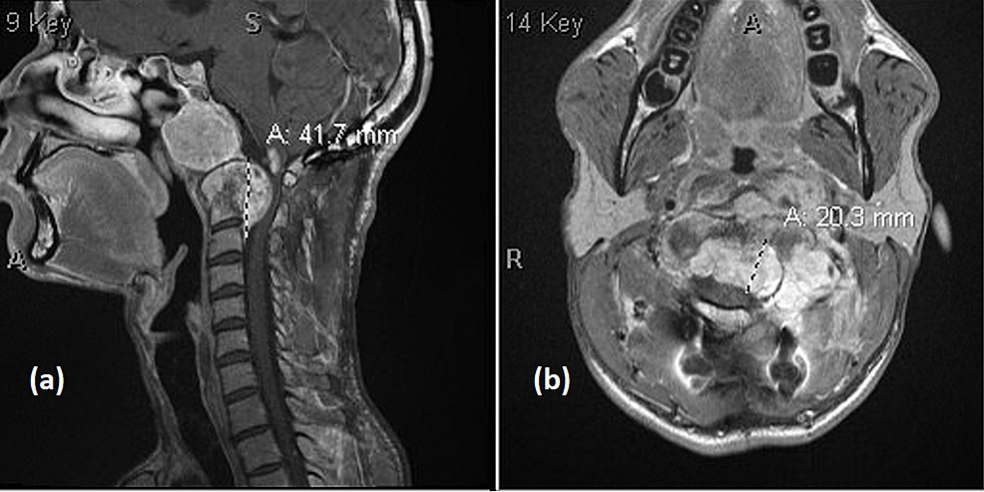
MRI spine of (a) large clival and (b) skull base chordoma. The image is showing large clival and skull base chordoma involving C1 and C2 with intraspinal epidural extension down to C3 and involvement of the left greater than right C1-C2 neural foramina. The mass effect on the cervicomedullary junction is greater in the left ventral cord than in the right.
